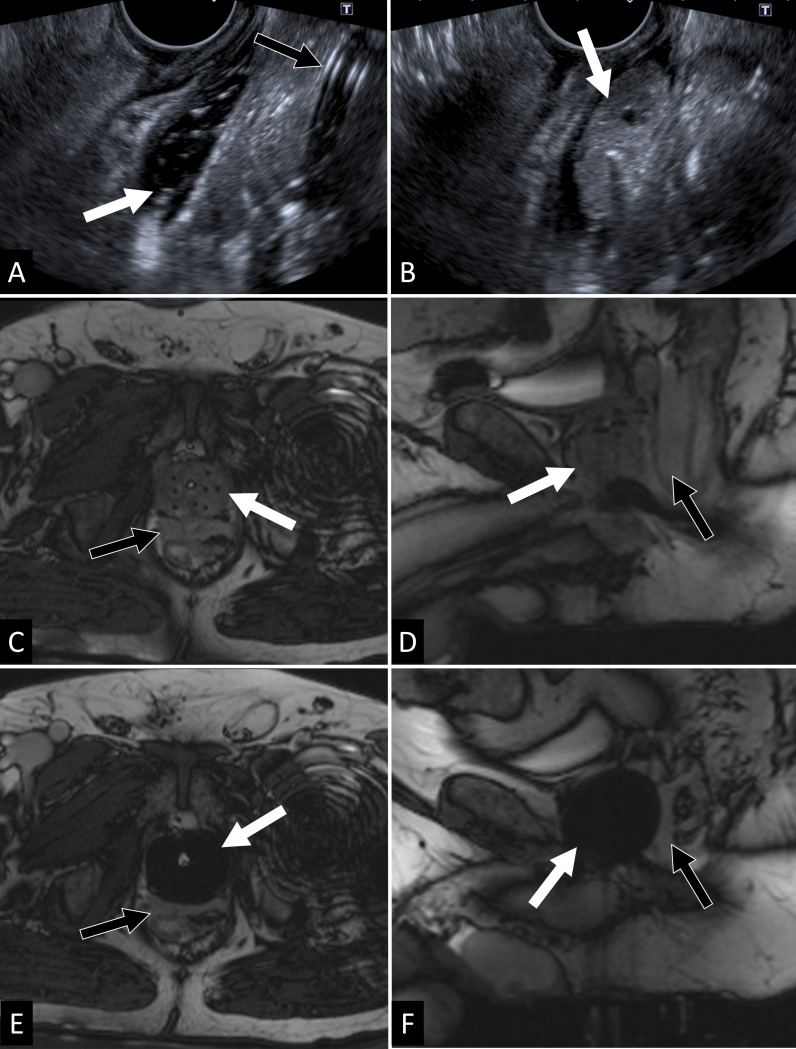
MR-guided whole gland CA as a primary treatment in a patient with a multifocal Gleason 4 + 3 PCa. A transperineal hemodissection of the Dennonvillier’s fascia is firstly performed under transrectal ultrasound guidance (A, B). The midline is repaired thanks to visualization of the urinary probe (black arrow in A). First some saline is injected to confirm the good positioning of the needle (white arrow showing the anechoic area in A). Afterwards autologous blood injection allows formation of a clot (arrow in B). Axial and sagittal *T*
